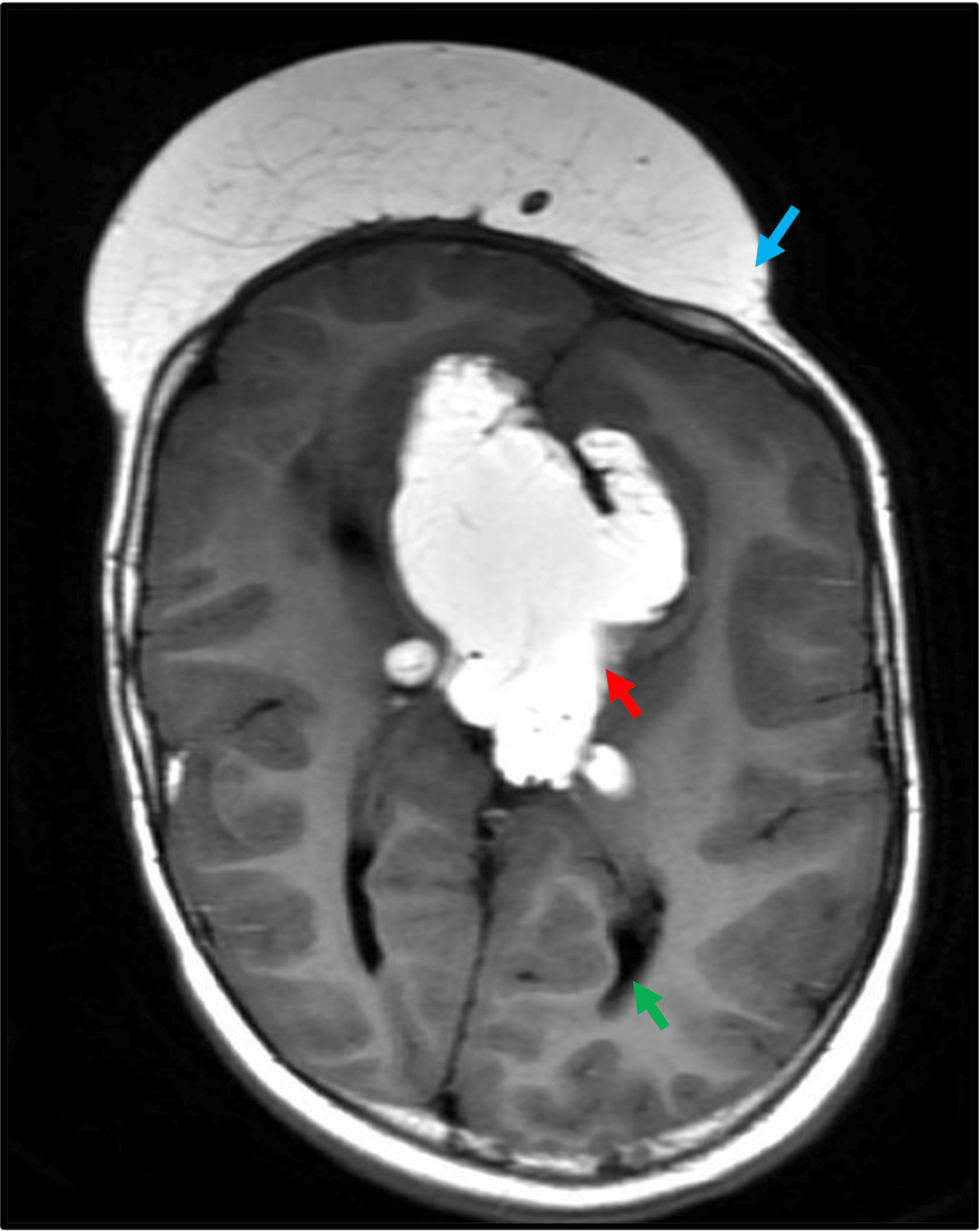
Corpus callosum agenesis with interhemispheric lipoma. Axial T1-weighted imaging showing lobulated T1 hyperintense interhemispheric lesion (red arrow). Note the parallel arrangement of lateral ventricles (green arrow). Note the subcutaneous frontal lipoma (blue arrow).
